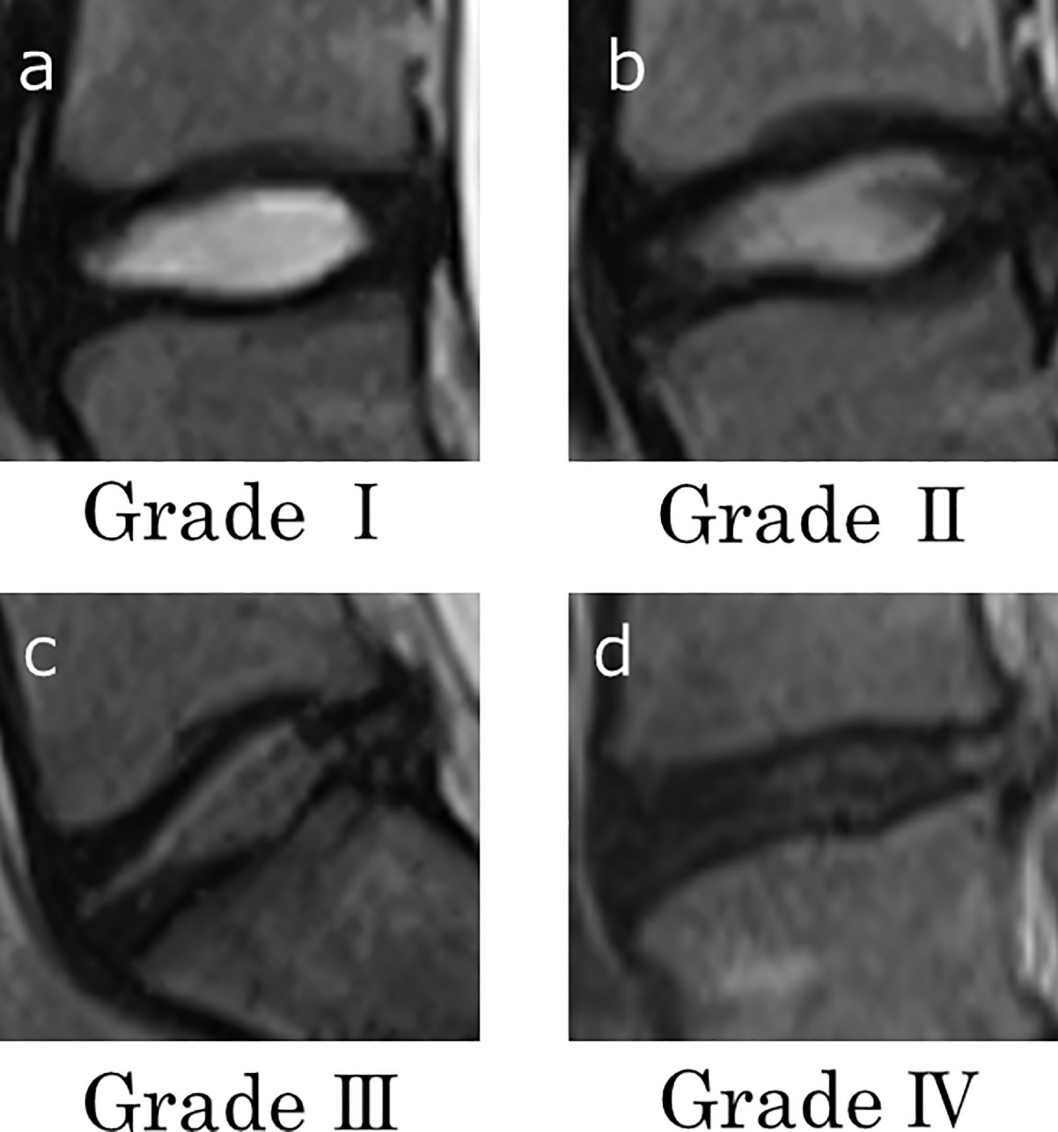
Standard T2-weighted MRI sagittal images of each Pfirrmann grade. We defined the Pfirrmann grade at each level of the lumbar vertebrae. Focused on the structure of the disc and disc height, it divided into grade Ⅰ(a), Ⅱ(b), Ⅲ(c), and Ⅳ(d), but grade Ⅳ was not absent in this study.
